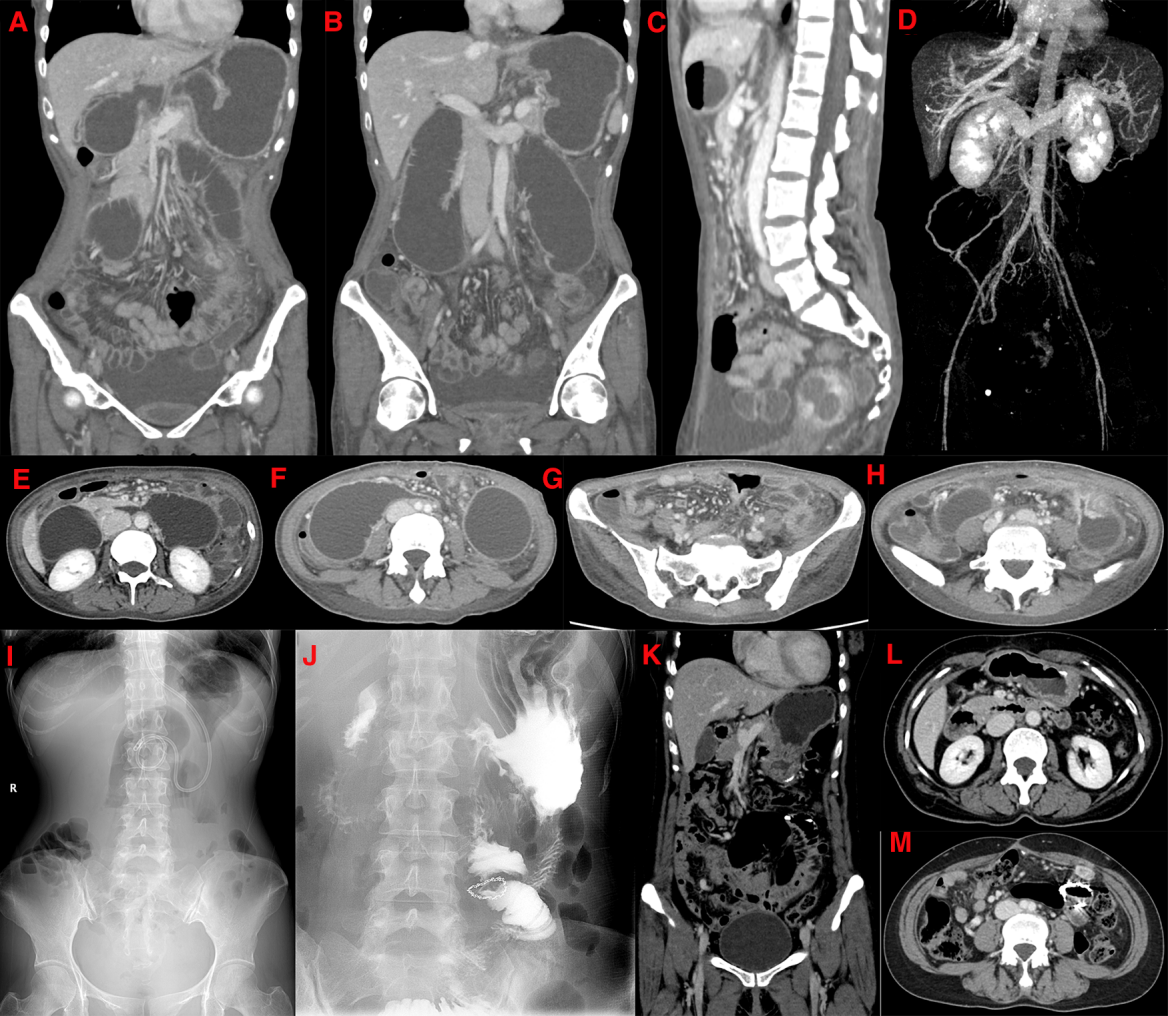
(May 22, 2017) CT examination of the patient's third admission. The coronal view shows a filling defect in the lumen at the origin of the linear filling defect in the superior mesenteric vein (A,B). The sagittal view shows a reduced angle between the superior mesenteric artery and the abdominal aorta (C). Maximal intensity projection shows a localized widening of the superior mesenteric vein with a hypodense filling defect (D). The axial view shows the filling defect sign of the superior mesenteric vein, a marked dilation of the horizontal segment of the duodenum and proximal jejunum, and a local constriction of the intestinal lumen near the origin of the superior mesenteric artery (E). The horizontal section of the duodenum is markedly expanded in the transverse axis, with a beak-like narrowing in the near midline area, and the proximal end of the ascending colon is also significantly expanded (F). The transverse axis shows inflammatory thickening and edema of the small intestinal wall at multiple sites, with a little mesentery, exudation and local thickening of blood vessels (G). Local small bowel dilatation in the left lower abdomen, with local wall thickening and enhancement, and the corresponding organ cavity is narrowing (H). (I): Preoperative upright abdominal plain film shows multiple lesions in the middle, and lower abdomen gas and liquid levels are seen, and the elastic ring sign is seen in a part of the dilated bowel. (J): Postoperative re-examination of the upright abdominal plain film shows changes after a part of the small bowel was resected. A CT scan after resection shows postoperative changes in the small bowel in the coronal view, and no signs of intestinal obstruction are found (K). After treatment, there is a little punctate filling defect sign in the superior mesenteric vein, which is more absorbed than before (L,M).
